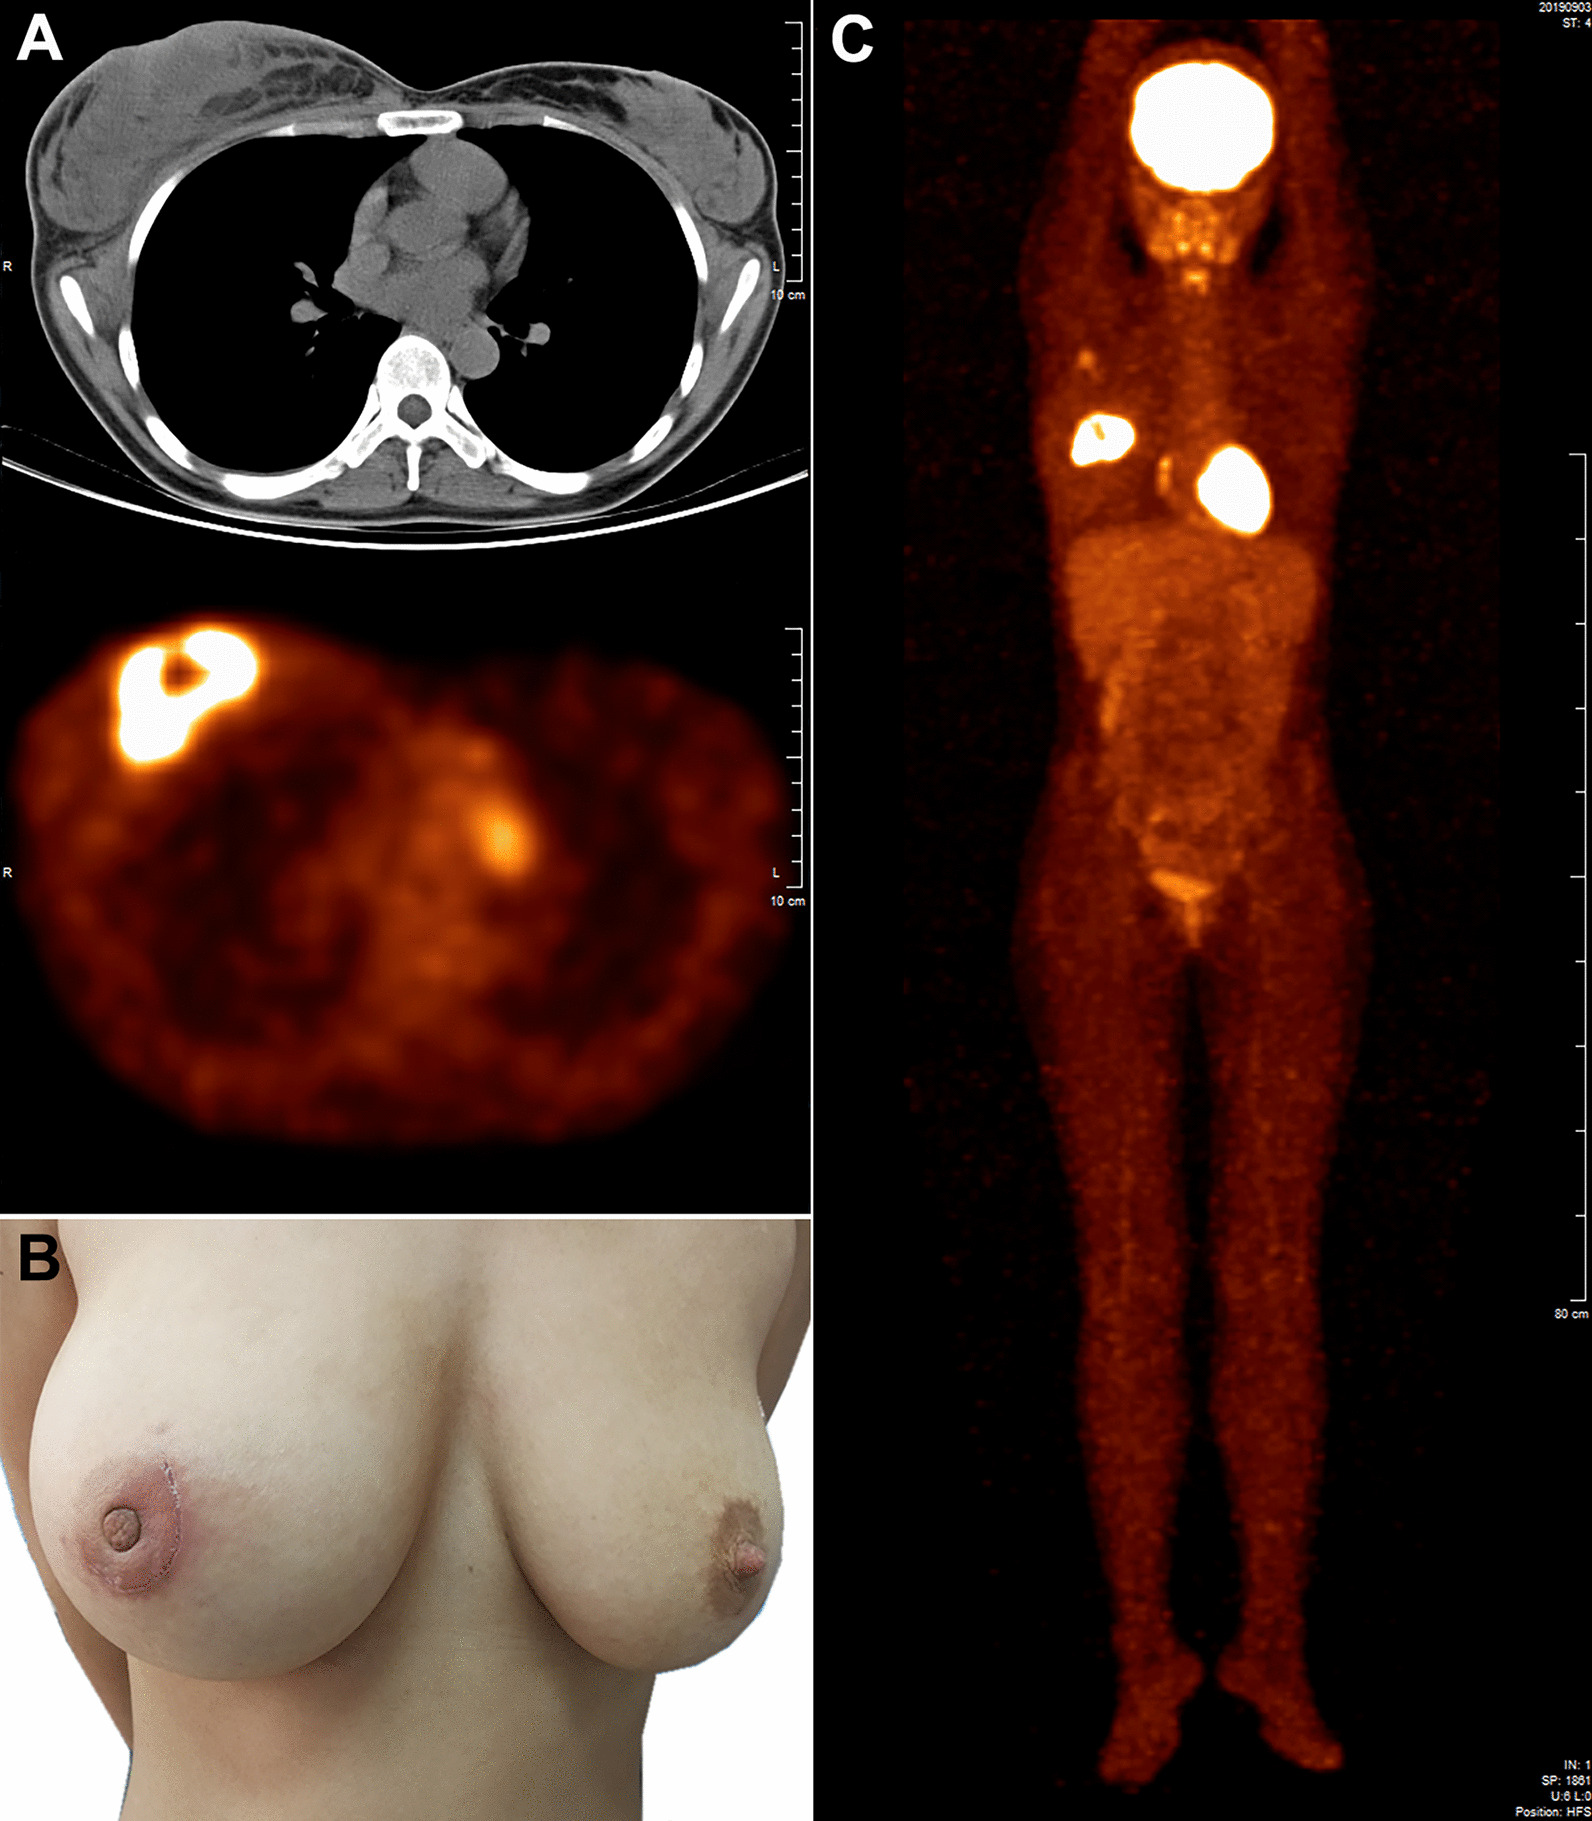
Clinical and radiological features of ENKTL-Breast (Case 1). a A CT scan showed that the lesion presented as a soft tissue density mass with a diameter of 6.6 cm in the right breast with unclear boundaries. b The right breast was significantly swollen and enlarged, and the nipple was indented. The mass located below the areola was partially excised for diagnosis (surgical incisions are apparent around the areola). c PET-CT showed increased F-FDG uptake by the breast lesion and ipsilateral axillary lymph node (stage IE)
